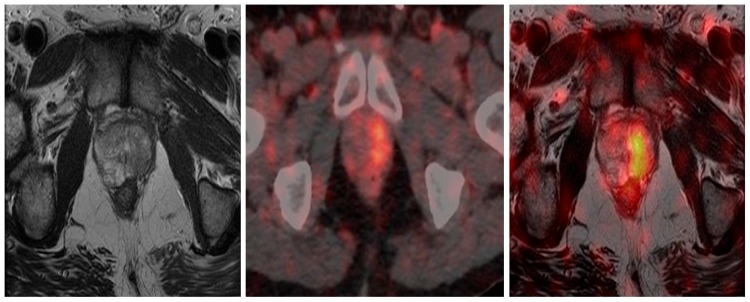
Primary prostate cancer. PIRADS 4/5 left lobe of prostate on multiparametric MRI. Fused to ^68^Ga-PSMA PET/CT images for MRI in-bore guided targeted biopsy.
